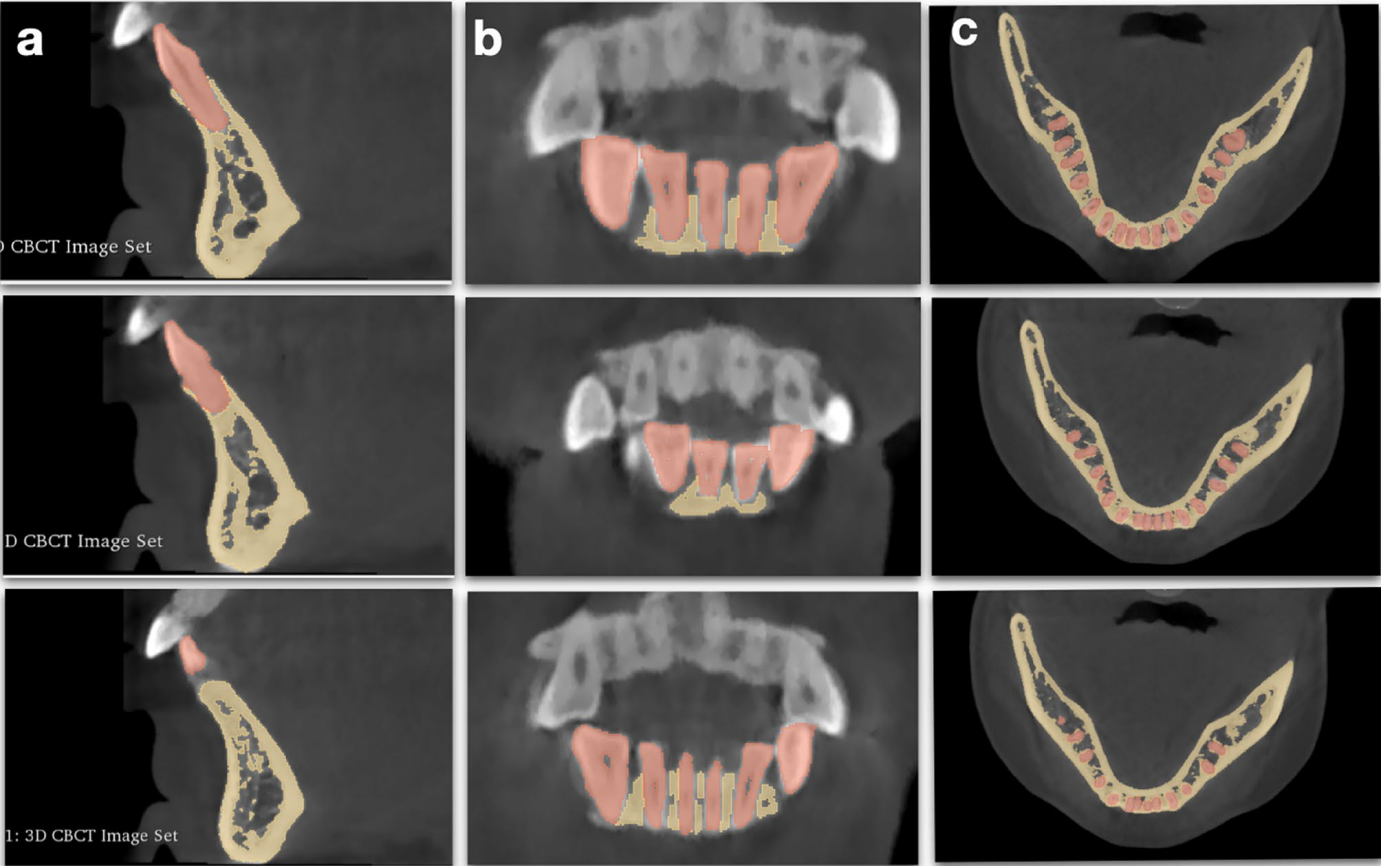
Regions of interest (ROIs) highlighted on slices of the CBCT dataset in 3D slicer software (Yellow: mandible; Orange: teeth). (a) Sagittal view. (b) Coronal view. (c) Axial view.
